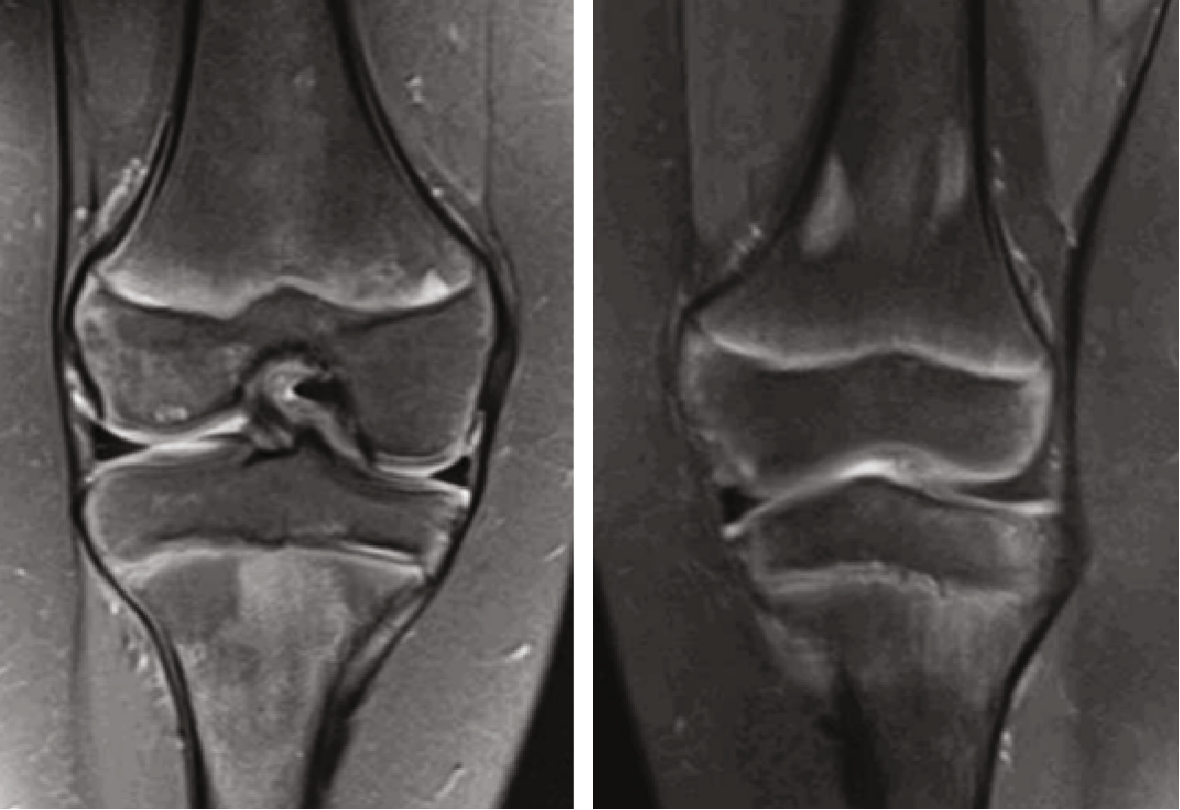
MRI of bilateral distal femora, PD TSE FS sequence, coronal view, demonstrating serpentine line of abnormal signal intensity with surrounding edema in subcortical bone of the lateral femoral condyle, consistent with avascular necrosis.
